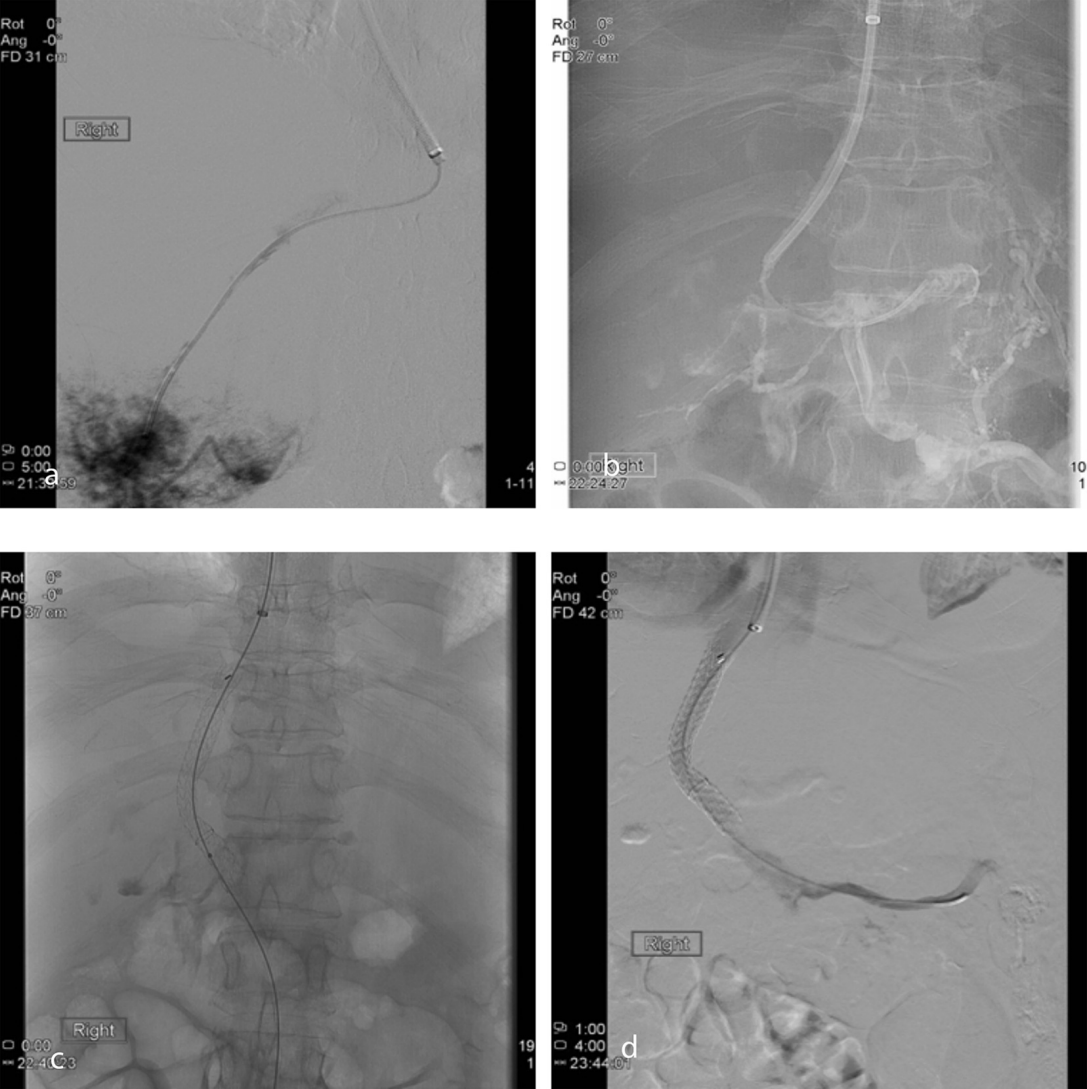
Images taken during the procedure demonstrating initial hepatic wedge venogram with filling defects within an occluded hepatic vein (a). Puncture of right PV and venogram demonstrated extensive occlusive thrombus in splenic vein, superior mesenteric vein, and main PV, confirming the CT scan findings (b). Following tract dilatation, a transjugular intrahepatic portosystemic shunt stent graft was successfully placed (c), with the venogram showing good flow through the stent graft but non-occlusive thrombus in the main PV (d). PV, portal vein.
